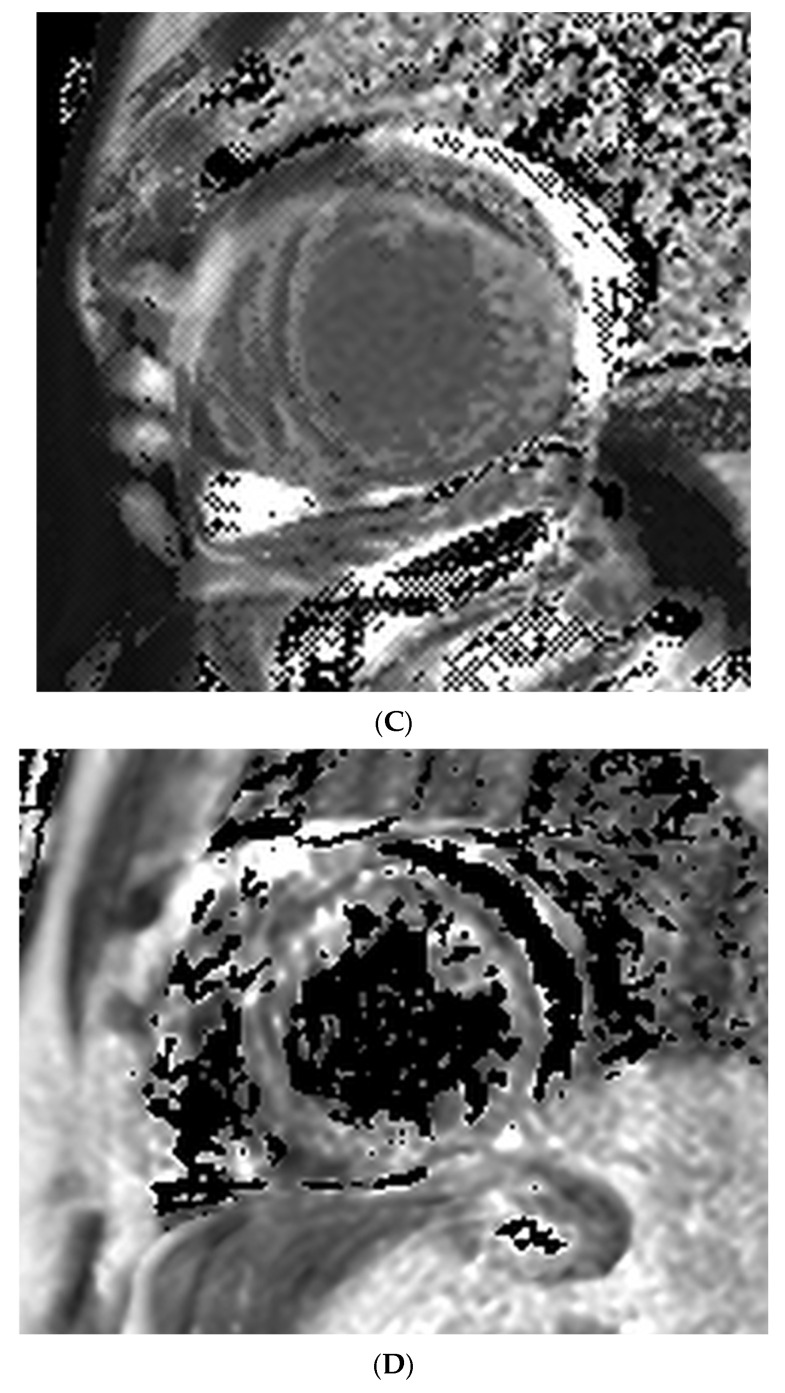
(A) Cardiac MRI LGE images from the clinical patient. Cardiac MRI images showing linear midventricular LGE (red arrows) in the basal and mid interventricular septum, extending into the anterior and inferior LV segments. Globally reduced biventricular function (LVEF 29% and RVEF 42%). (B) Cardiac MRI T2-weighted images from the clinical patient. Cardiac MRI T2-weighted images. There is interventricular T2 enhancement (red arrow) consistent with edema. (C) Cardiac MRI T1 mapping images from the clinical patient. Cardiac MRI T1 mapping images revealed an elevated native T1 map at 1470 ms (normal 1000 +/− 50 ms at 1.5T). (D) Cardiac MRI T2 mapping images from the clinical patient. Cardiac MRI T2 mapping images revealed elevated T2 mapping at 70 ms (myocardial edema suggested if T2 > 55–60 ms).
